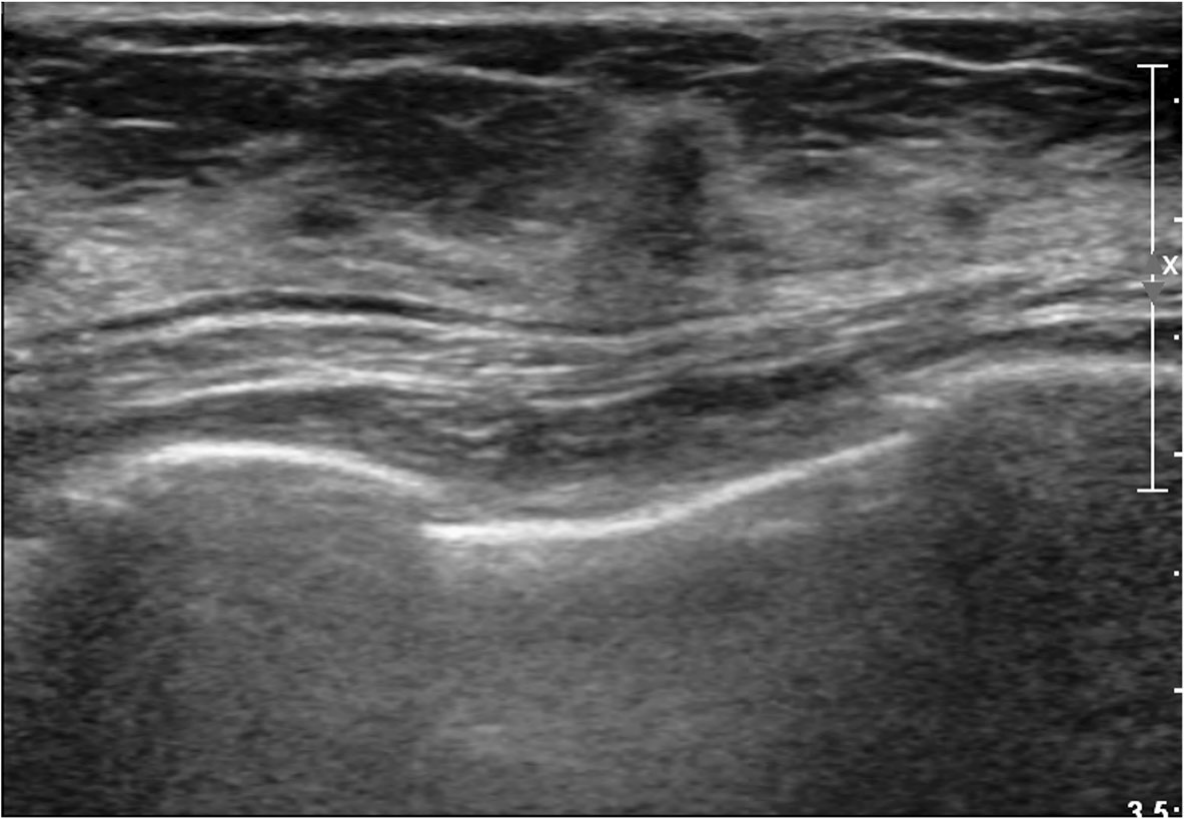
A radial scar on ultrasound- guided core needle biopsy and upgraded to ductal carcinoma in situ after surgical excision. Ultrasound shows an irregular, non-parallel, indistinct, and hypoechoic mass
